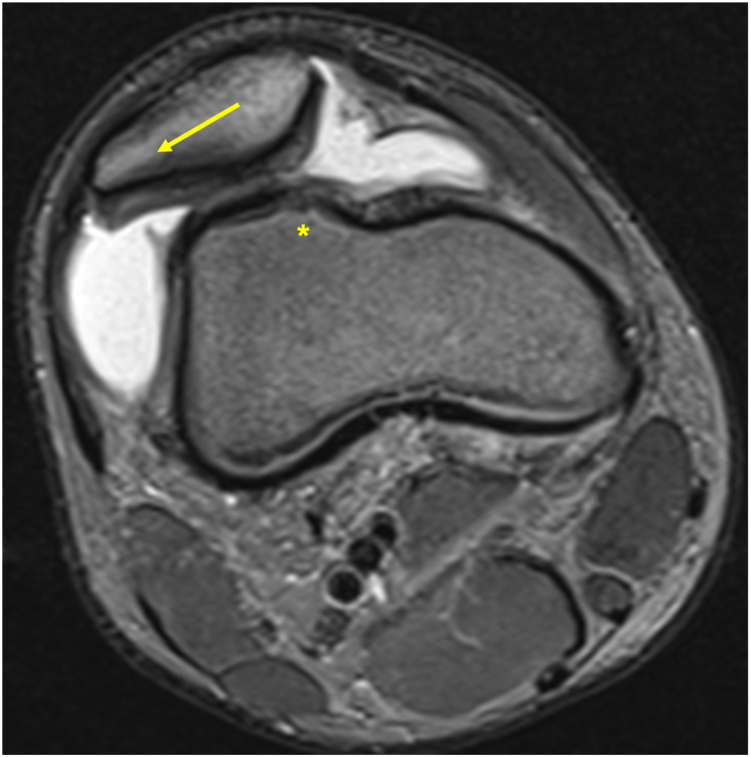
Axial magnetic resonance imaging of a right knee with chronic patellofemoral instability, lateral maltracking of the patella (yellow arrow) with tightness of the lateral retinaculum, medial patellofemoral ligament insufficiency and high grade trochlea dysplasia (∗).
